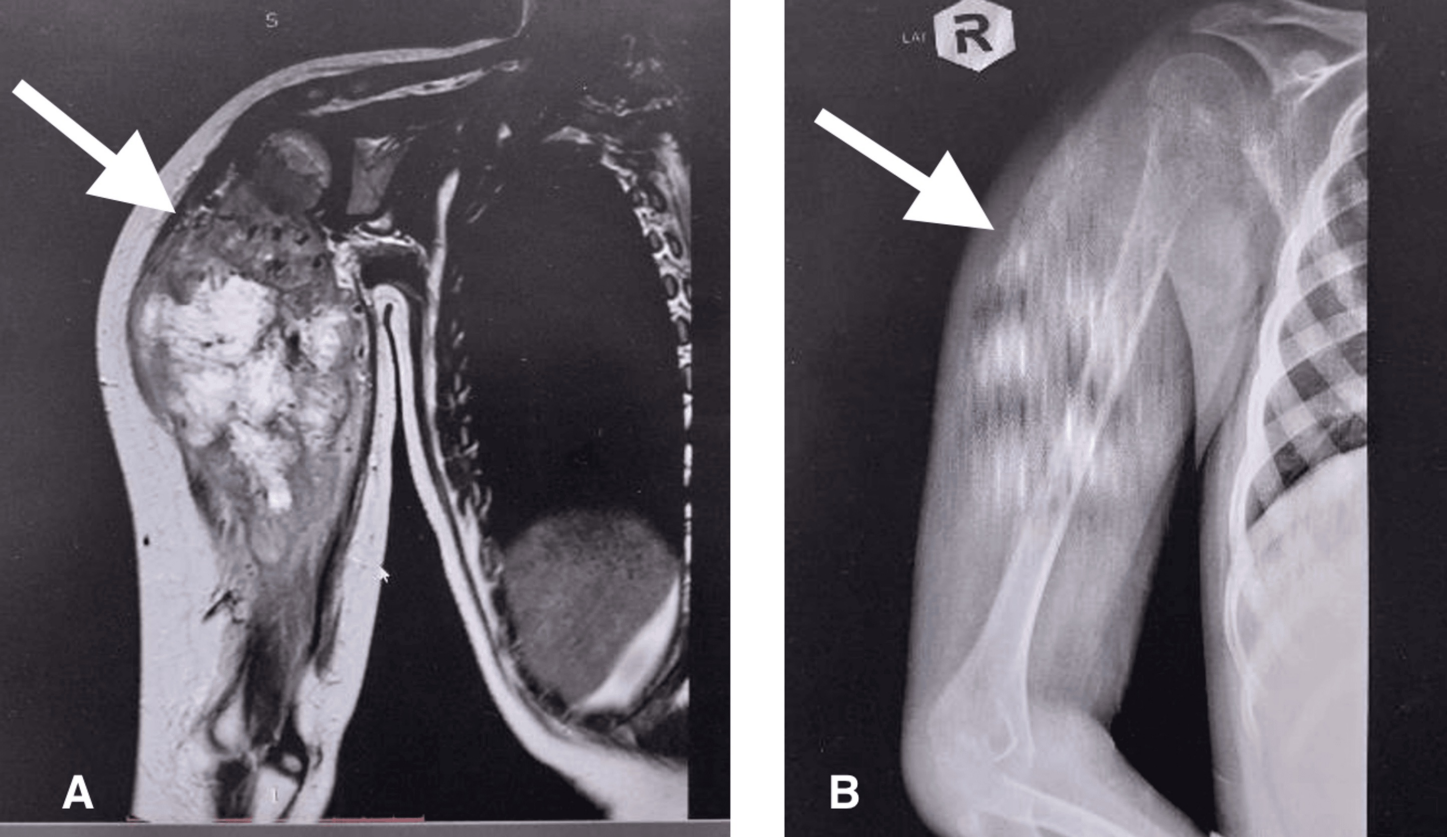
Preoperative (A) MRI T2-weighted image (coronal view) and (B) X-ray of a 14-year-old male patient showing the tumour (white arrow)
